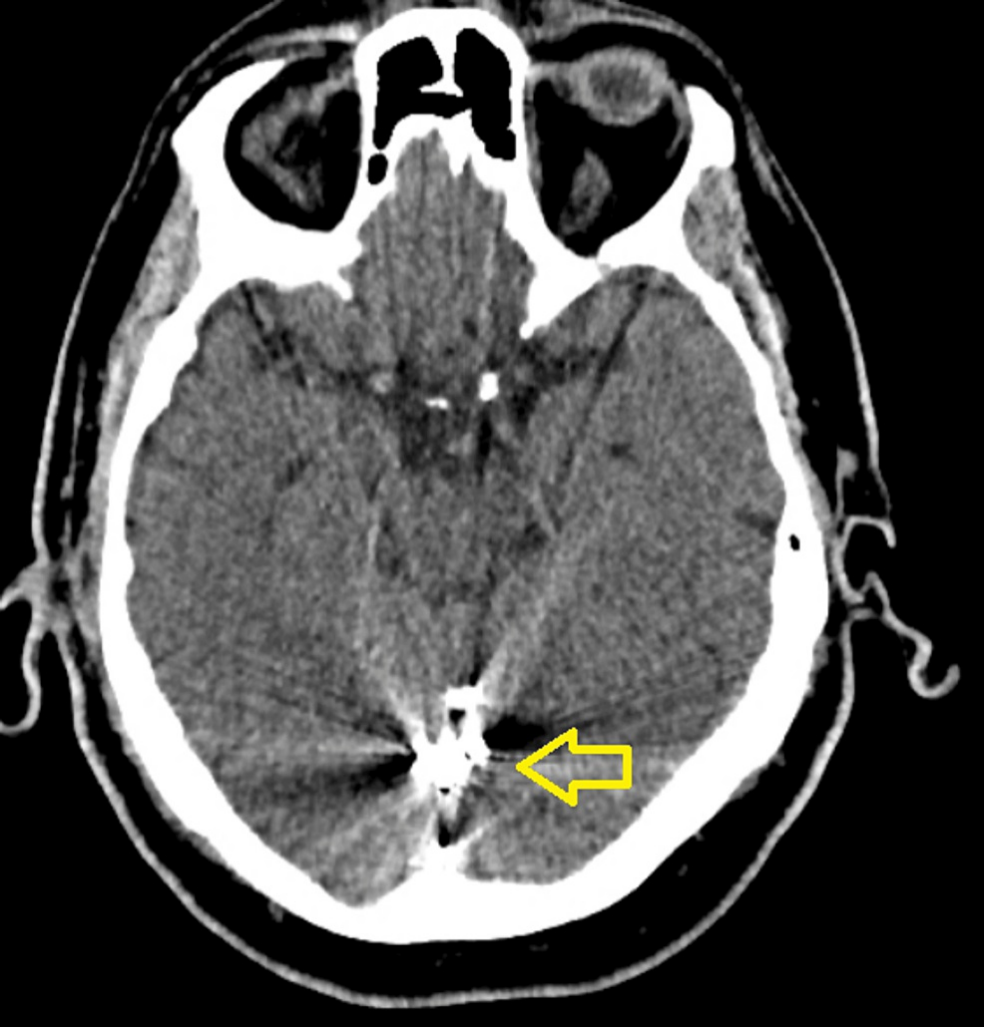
An axial cut of the non-contrast CT head, with an arrow pointing to the dense Onyx cast on the posterior cranial fossa. CT, computed tomography
